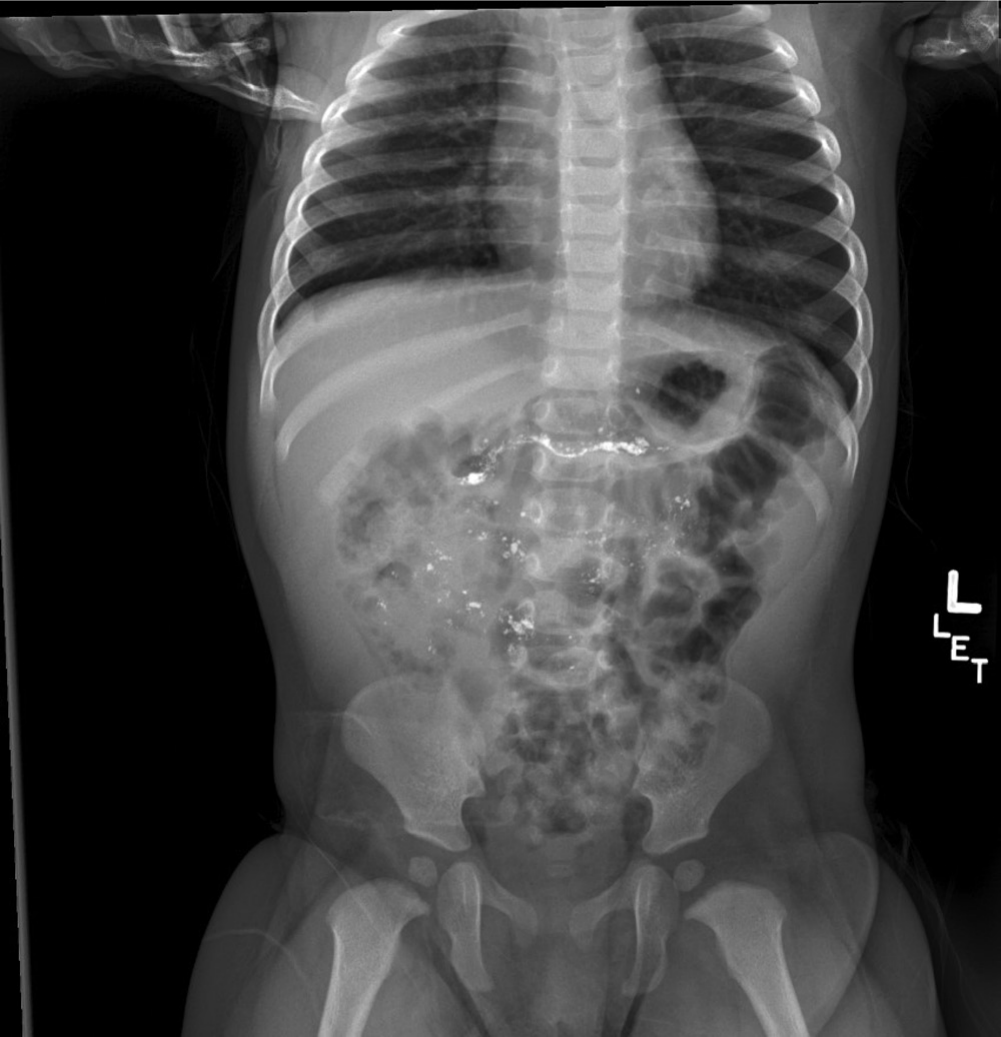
Abdominal radiograph approximately 2 hours after ingestion demonstrating hyperdense material in the proximal small intestine.
